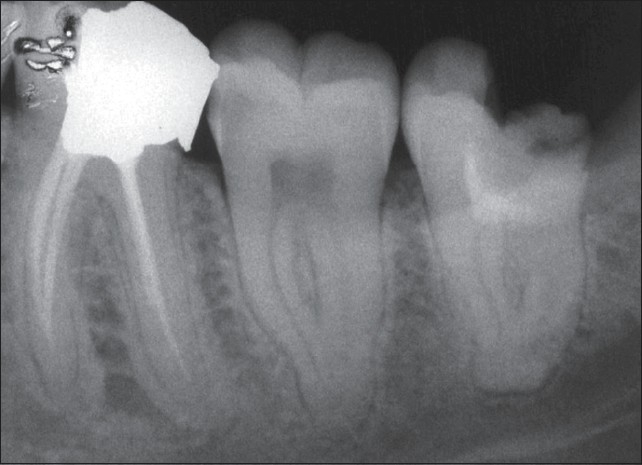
Two-month follow-up radiograph showing pulpotomized tooth without coronal filling. The patient had extracted the tooth because it was not restorable
